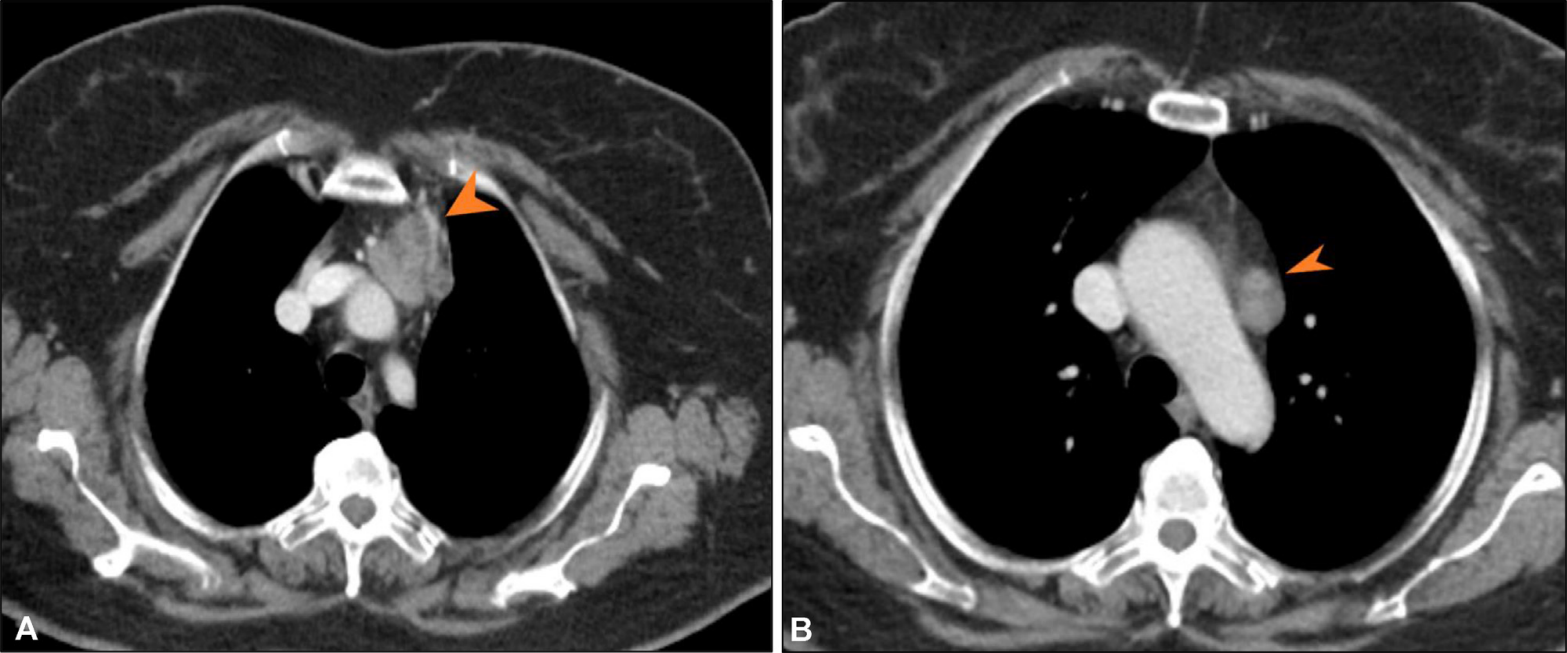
CT scan chest axial cuts (A and B) with soft tissue window show interval enlargement of mediastinal lymph nodes (arrowheads).
